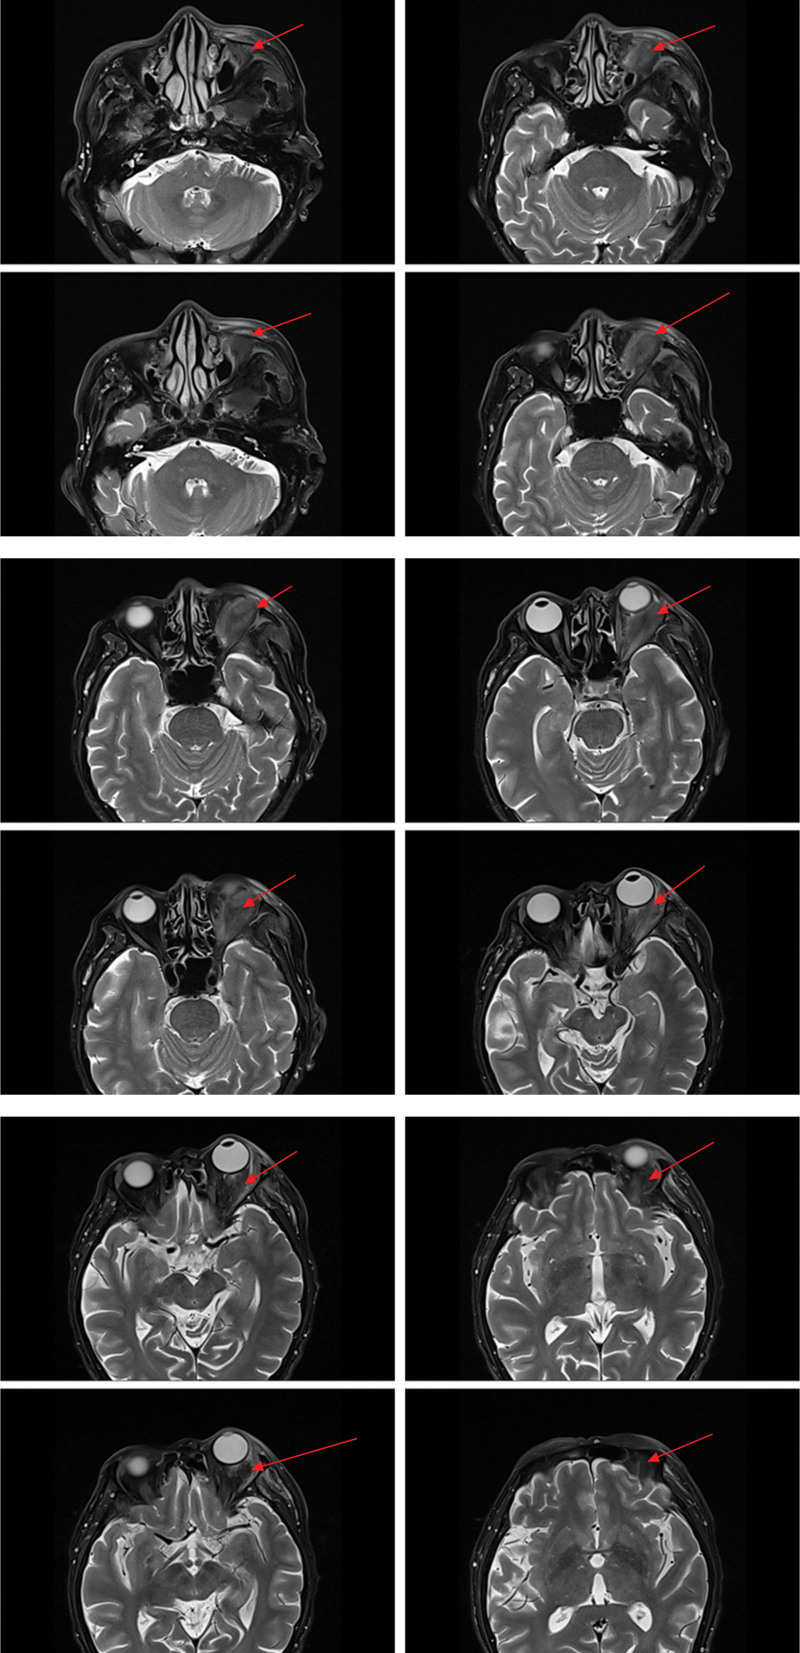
The patient MRI cross-sectional T2 phase showed slightly high signal without significant enhancement of the left posterior fat space, left lateral eyeball, and left inferior muscle space masses (red arrows). MRI = magnetic resonance imaging.
